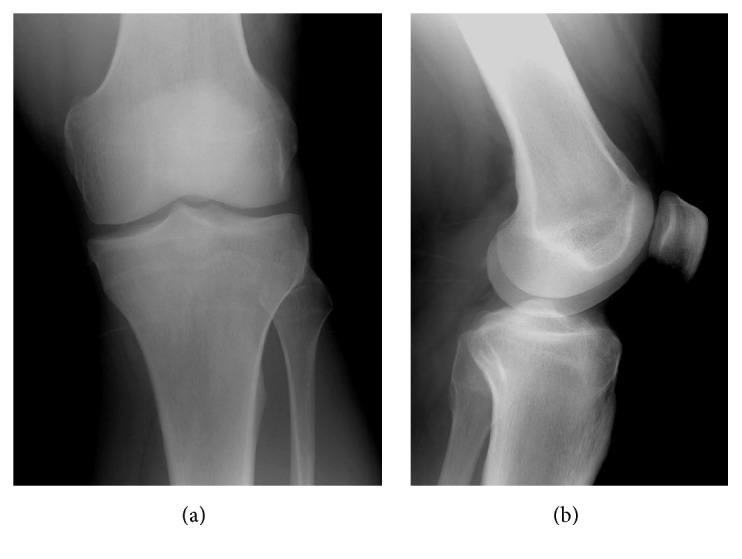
Normal findings at plain radiography.
